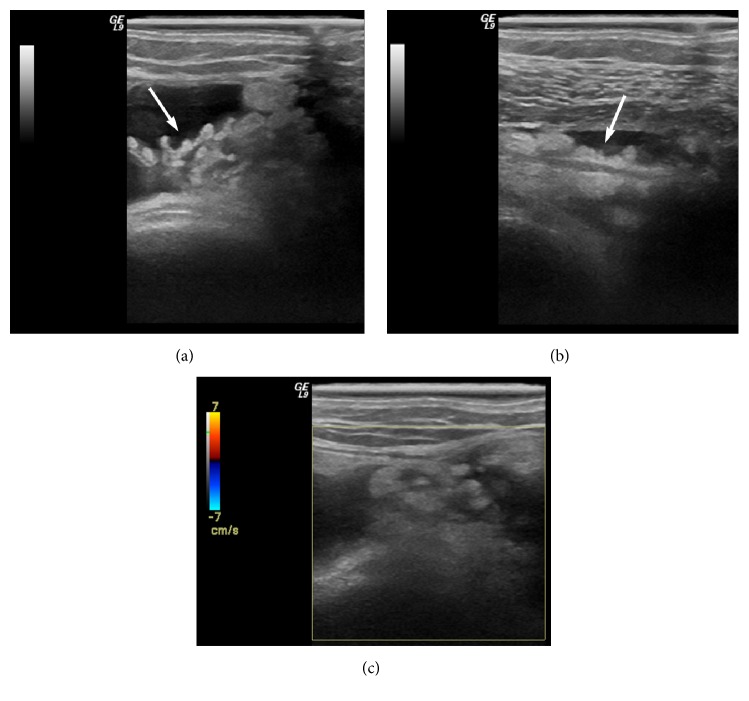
US examination of the right knee on axial (a) and longitudinal (b) sonograms reveals hyperechoic frond-like projections of the synovium into the effusion (arrows), findings suggestive of LA. Colour Doppler US (c) does not depict any blood flow in the affected area.
